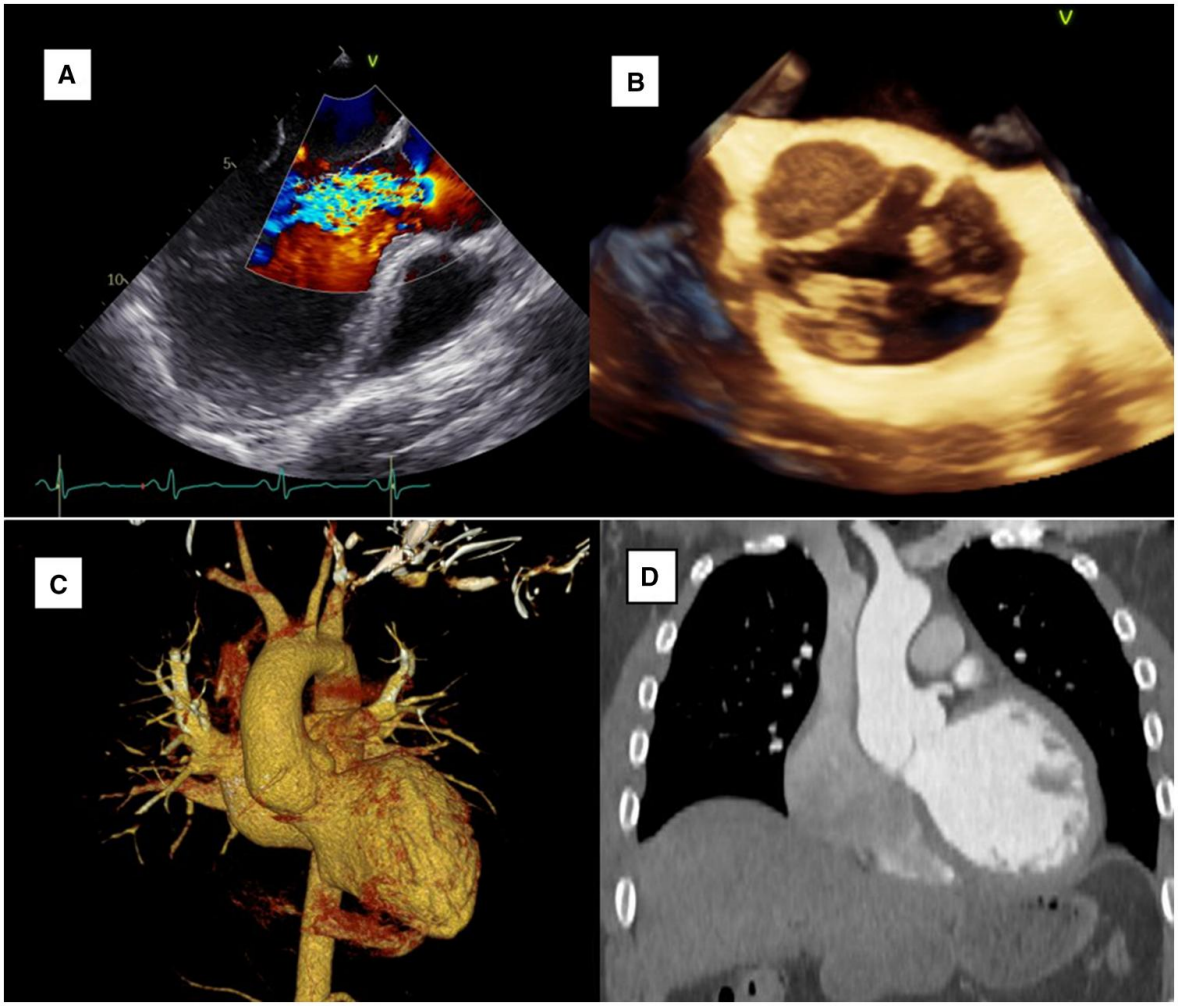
Preoperative Transoesophageal Echocardiography (TOE) and CT Imaging. (A, B) Mid-oesophageal long- and short-axis TOE showing severe eccentric aortic regurgitation and right–noncoronary commissural avulsion. (C, D) CT angiography confirming root dilation and excluding dissection.
